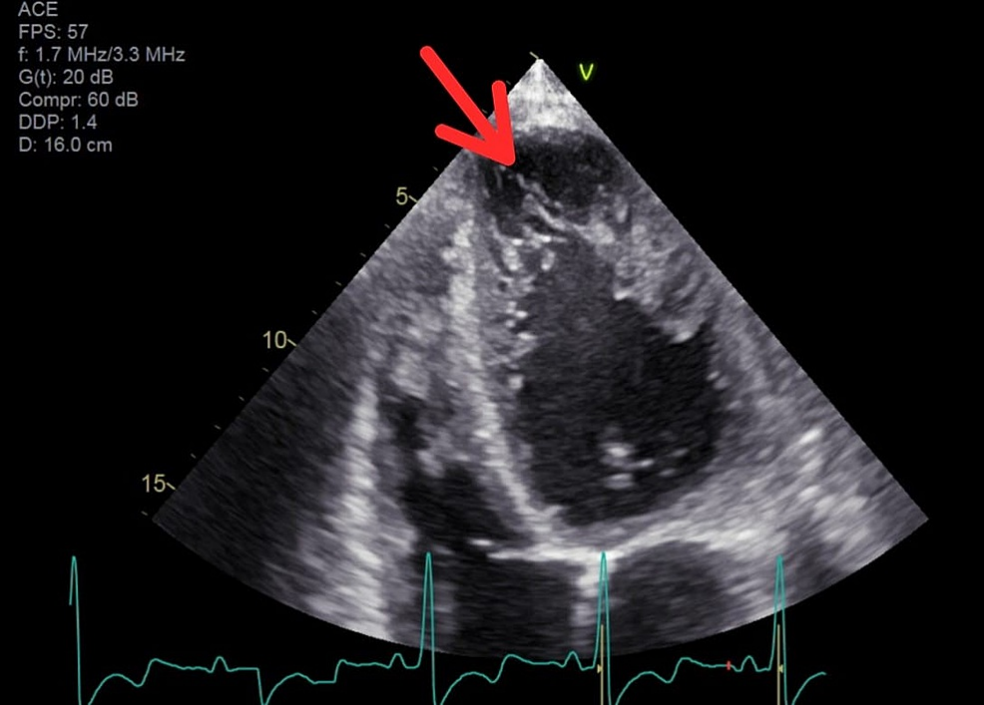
Apical four-chamber view on transthoracic echocardiography showing prominent left ventricular wall trabeculations The red arrow highlights the area of non-compacted left ventricular myocardium with trabeculations.
